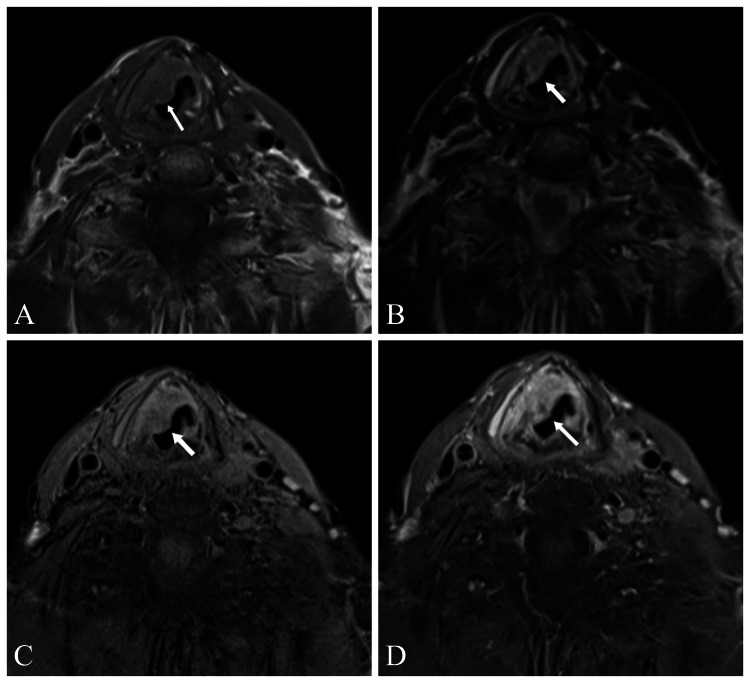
Contrast-enhanced neck MRI of the mass without fat suppression T1-weighted (A), without fat suppression T2-weighted (B), pre-contrast fat suppressed T1-weighted (C), and post-contrast fat-suppressed T1-weighted (D) A homogeneous mass lesion 23×15×12 mm in size (AP×TR×CC) was observed with lobulated contours, hypointense in T1-weighted, hyperintense and heterogeneous in T2-weighted, with homogeneous contrast enhancement in postcontrast T1-weighted fat-suppressed images (white arrows). AP: Anteroposterior, TR: Transverse, CC: Craniocaudal
